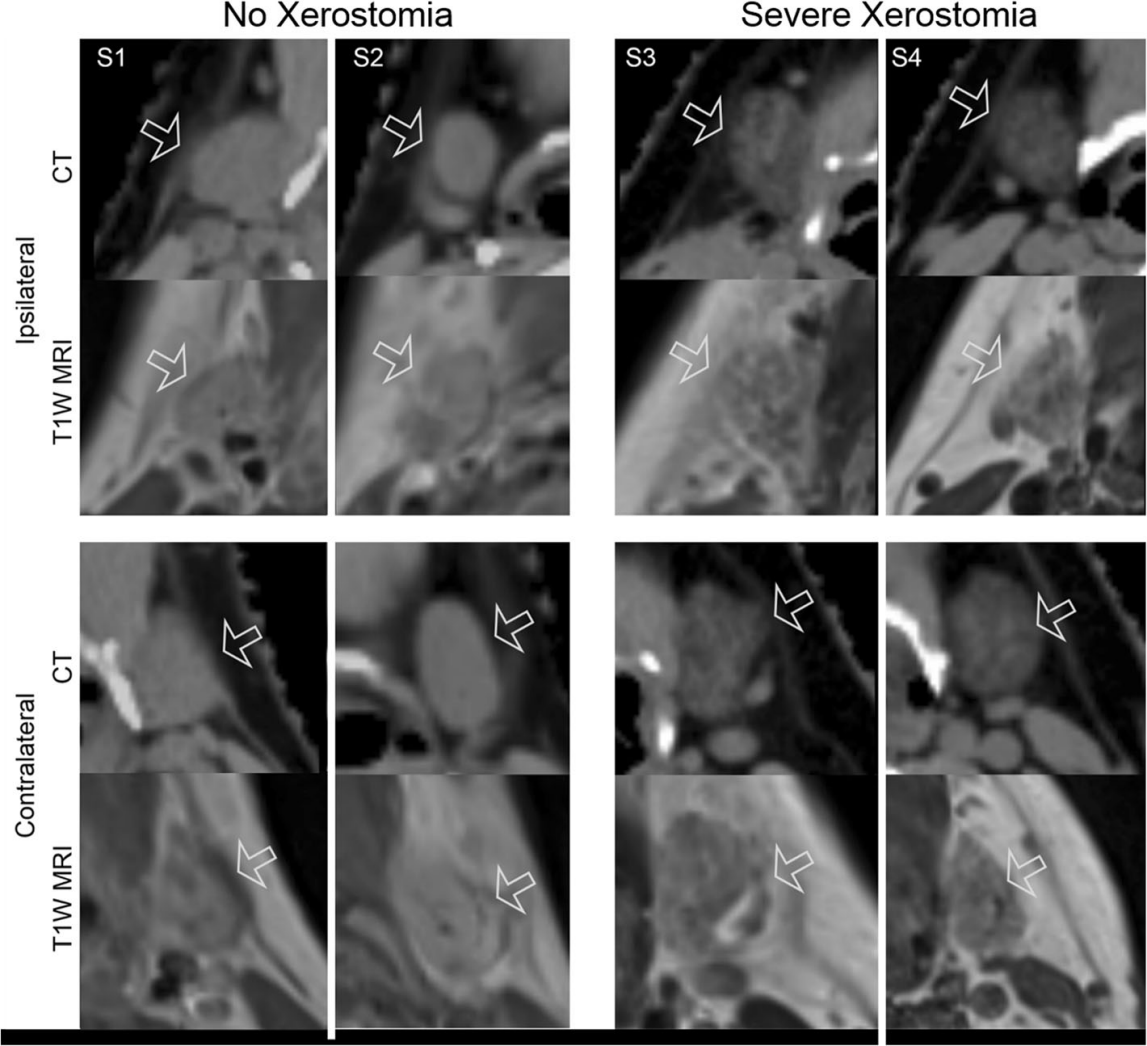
CT and MR images of representative patients’ submandibular glands. From left to right: S1: 71-yr old male with squamous cell carcinoma of the base of tongue, S2: 82-yr old female with melanoma of nasal cavity, S3: 54-yr old female with squamous cell carcinoma of the tonsil, and S4: 61-yr old male with squamous cell carcinoma of the nasopharynx. Images are displayed using the same window and level
